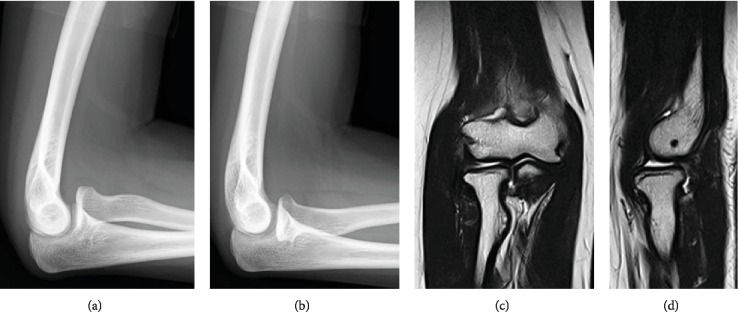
Radiographic images at 1-year follow-up. The lateral radiographs ((a) supination, (b) pronation) and T2-weighted magnetic resonance images ((c) coronal, (d) sagittal) show the reduced RH with reconstructed annular ligament.
